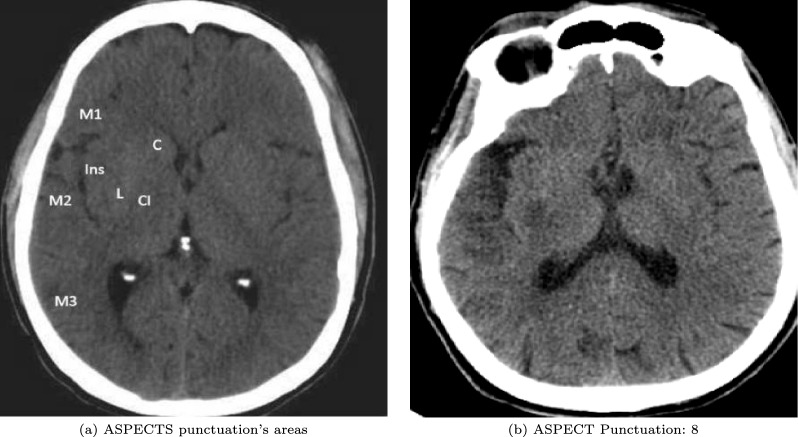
View of ASPECTS punctuation’s areas, detailing an ischemia in M2 and L areas
